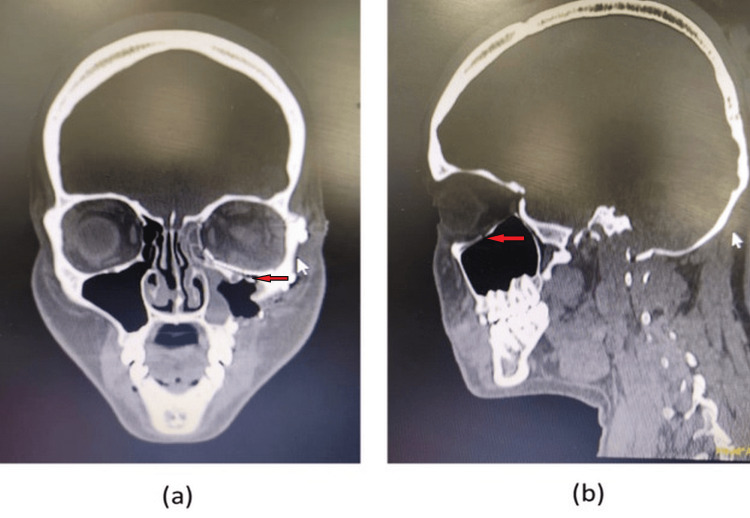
Postoperative CT images showing reduced and fixed orbital floor (a) Coronal view; (b) sagittal view
